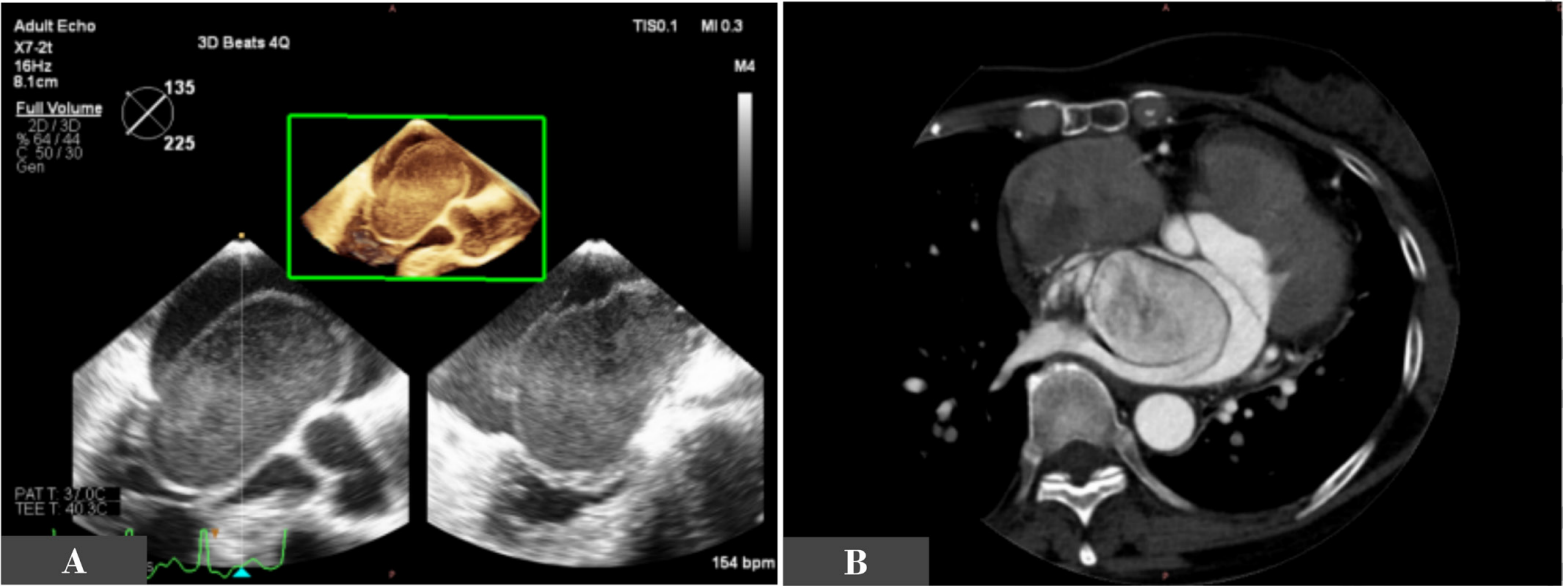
Preoperative three-dimensional transoesophageal echocardiography (A) and cardiac CT in the arterial phase (B).
